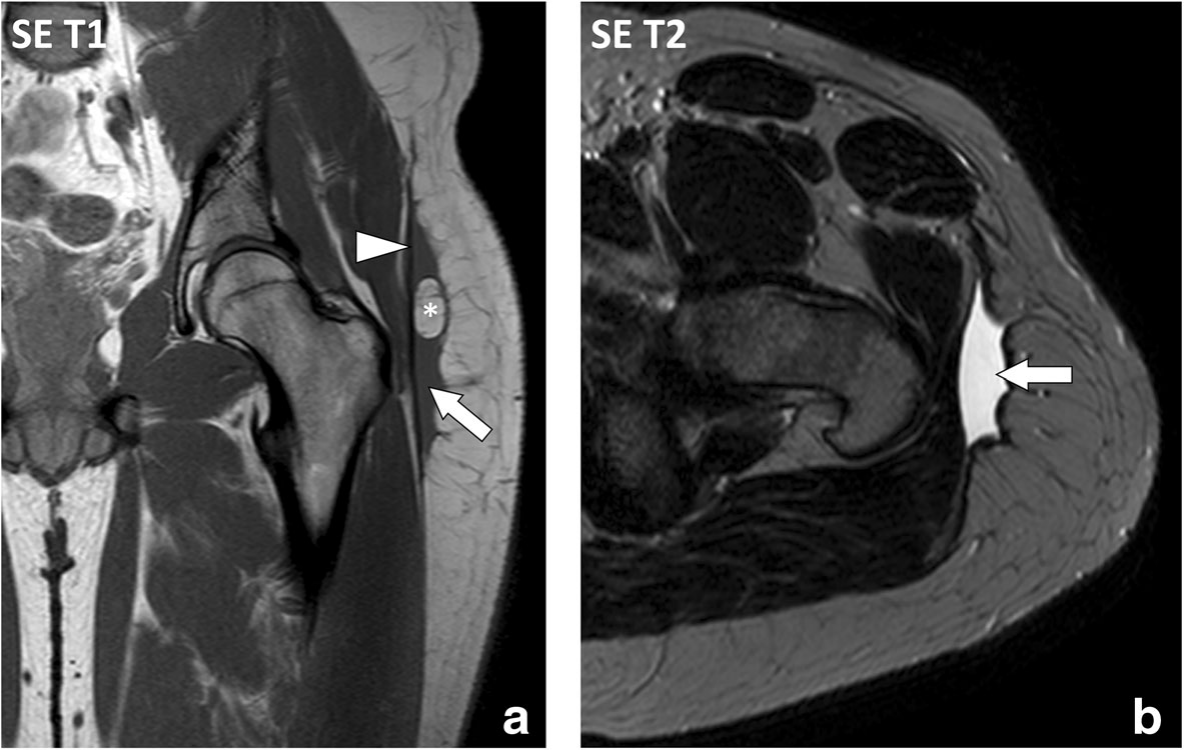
a Coronal SE T1-weighted (T1w) and (b) axial SE T2-weighted (T2w) images of the left hip and proximal part of the left thigh of a 22-year-old male with Morel-Lavallée lesion after a street fight. MRI demonstrates an extensive lenticular fluid collection (arrows) deep to the hypodermis and superficial to the fascia lata (arrowhead). Note the large fat lobule bulging into the collection (asterisk)
